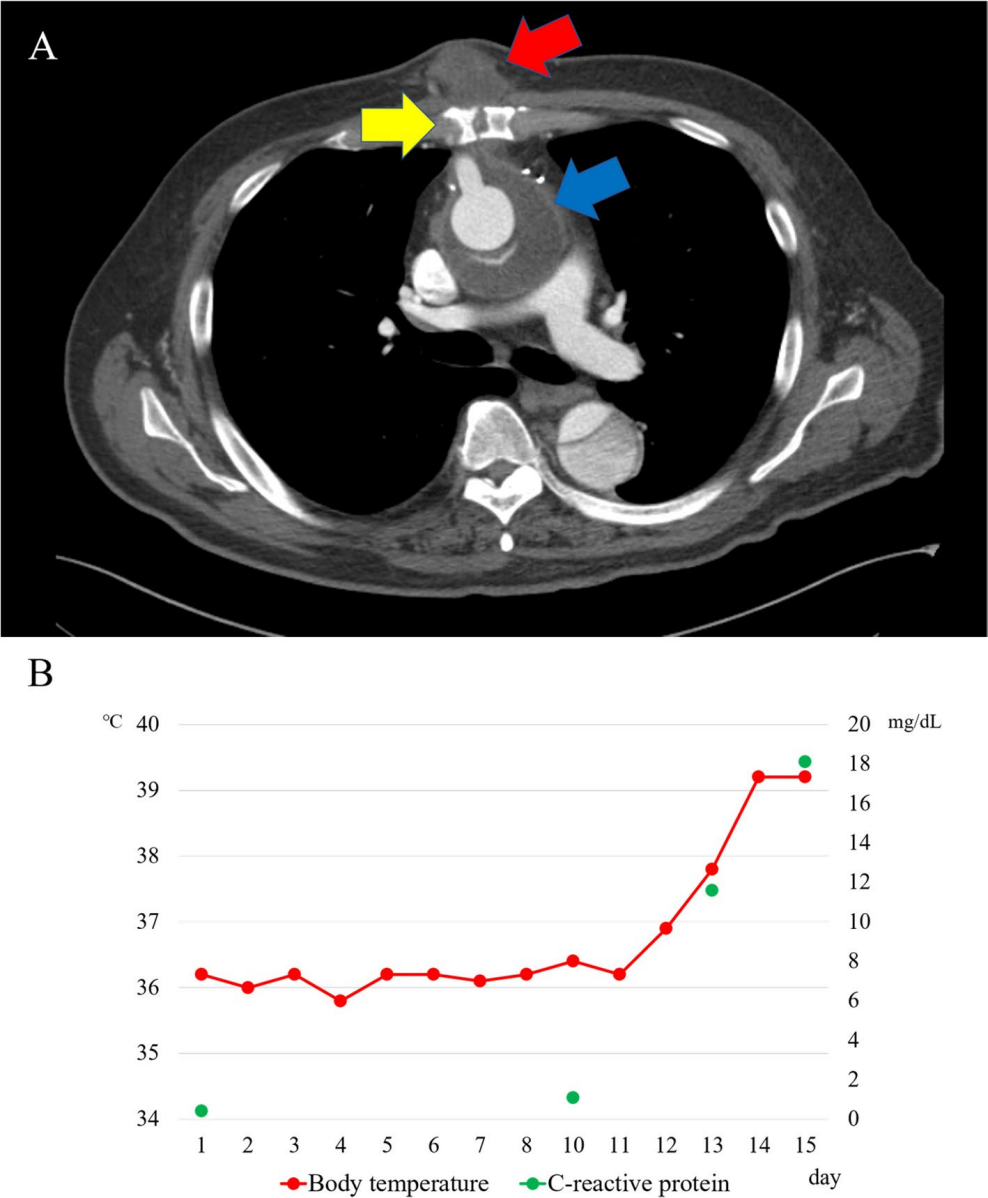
A Enhanced CT findings in case 1. Enhanced CT revealed a subcutaneous low-density mass (red arrow) sized 33 × 32 mm in the center of the median sternotomy, continuous with a 25-mm-wide area of fluid collection (blue arrow) around the ascending aortic graft with no extravasation of contrast media observed. The gap of the closed sternum was also visualized (yellow arrow). B Chart for case 1 patient body temperature and C-reactive protein level. Shown is the chart of body temperature (red point) and C-reactive protein levels (green point) recording during hospitalization. On day 12 after admission, a fever developed, and the creative protein level was drastically elevated to 11.2 mg/dL
